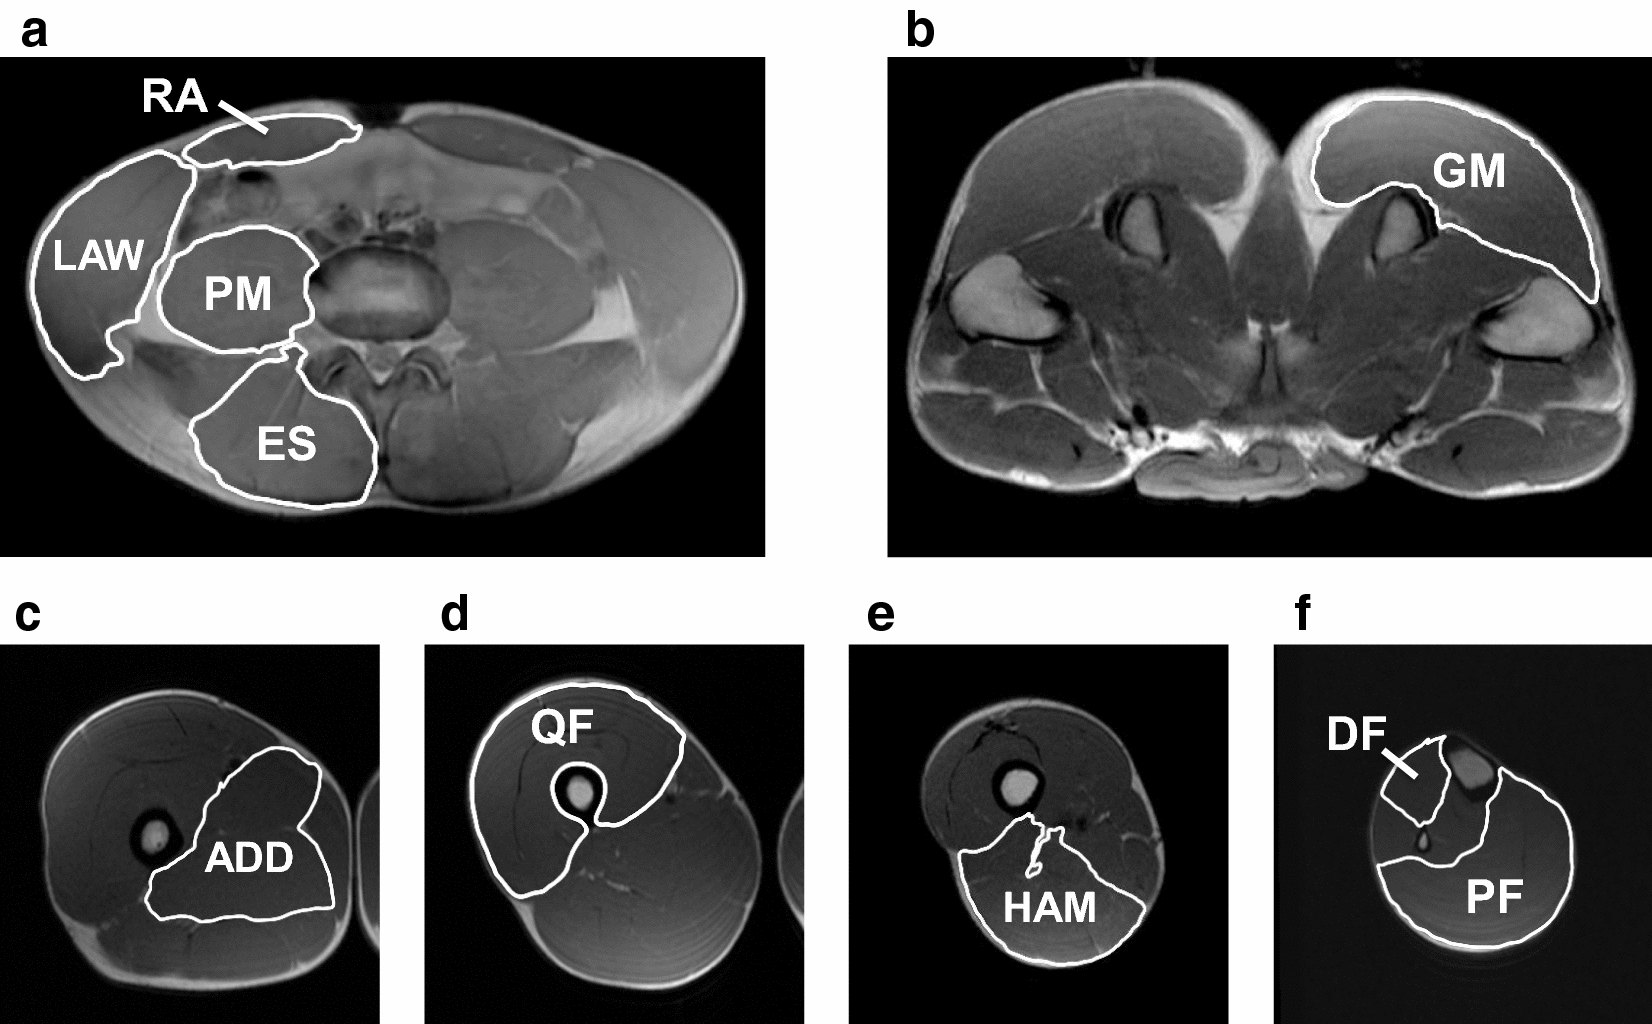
Representative magnetic resonance imaging scans for measuring cross-sectional areas of trunk and lower limb muscles. The cross-sectional areas (CSA; CSAs) of the rectus abdominis (RA), lateral abdominal wall (LAW), elector spinae (ES), and psoas major (PM) were obtained at the mid-level of the L4–L5. a The CSA of the gluteus major (GM) was obtained at the level of the greater trochanter. b The CSA of the adductors (ADD) was obtained at the proximal 30% of the thigh length. c The CSA of the quadriceps femoris (QF) was obtained at 50% of the thigh length. d The CSA of the hamstrings (HAM) was obtained at 70% of the thigh length. e The CSAs of the dorsiflexors (DF) and plantar flexors (PF) were obtained at the proximal 30% of the lower leg length (e)
